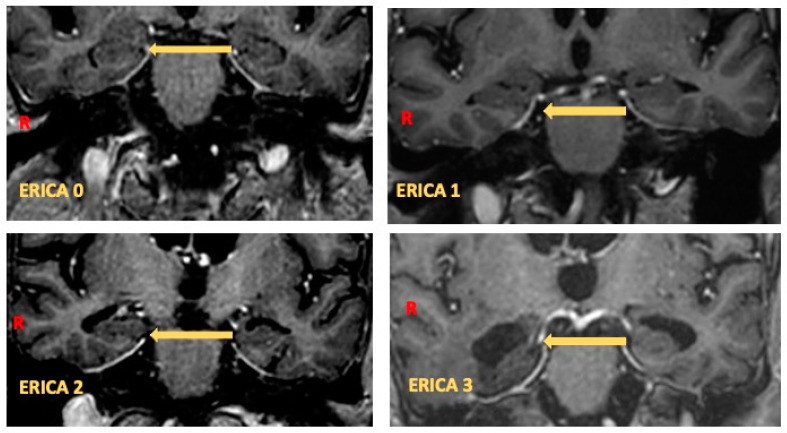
ERICA scores in coronal MRI slice: ERICA score 0, normal volume of entorhinal cortex and parahippocampal gyrus; ERICA score 1, mild atrophy with widening of collateral sulcus; ERICA score 2, moderate atrophy of entorhinal cortex with “tentorial cleft sign”; and ERICA score 3, emphatic atrophy of parahippocampal gyrus with wide cleft between entorhinal cortex and cerebellar tentorium.
